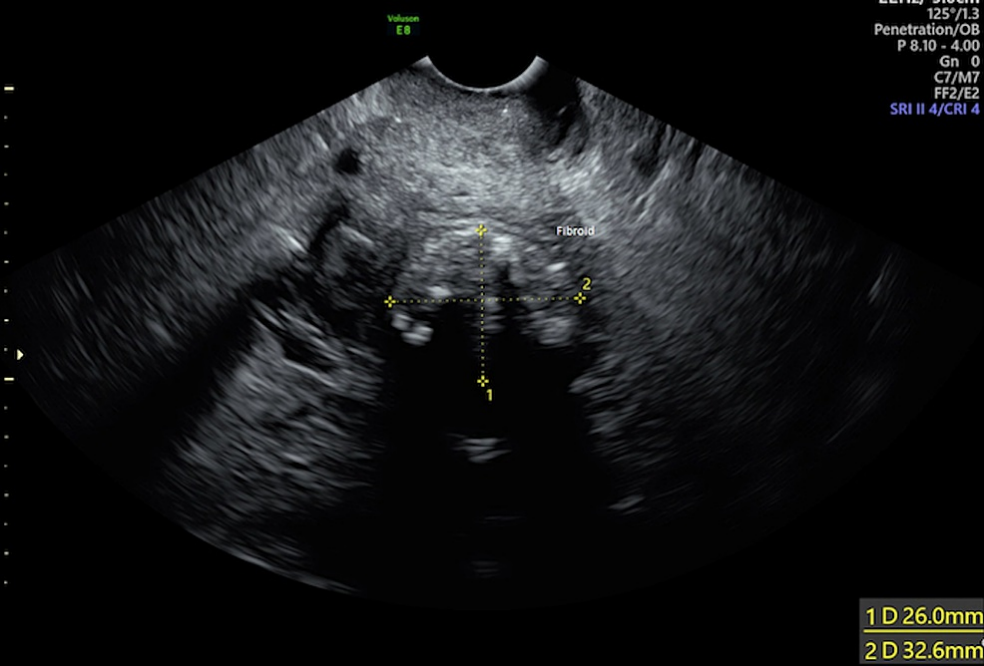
Ultrasound image displaying a small mass in the anterior myometrium measuring 3.2 × 2.6 × 3.4 cm, which represented a calcified fibroid.
